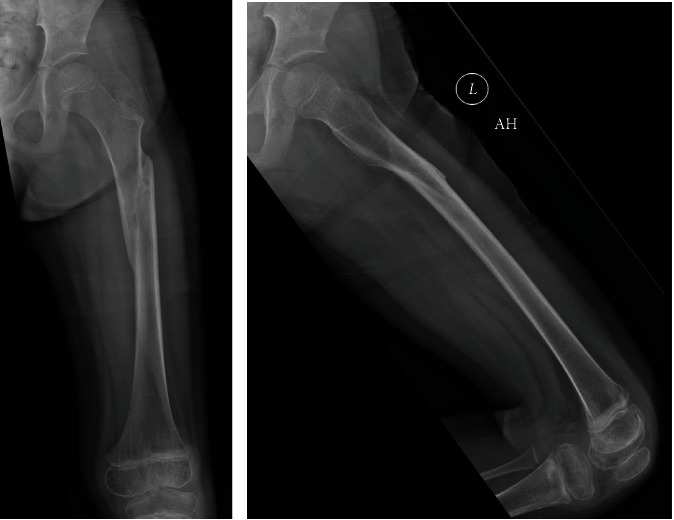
Healed X-rays from clinic 10 weeks after spica casting.
